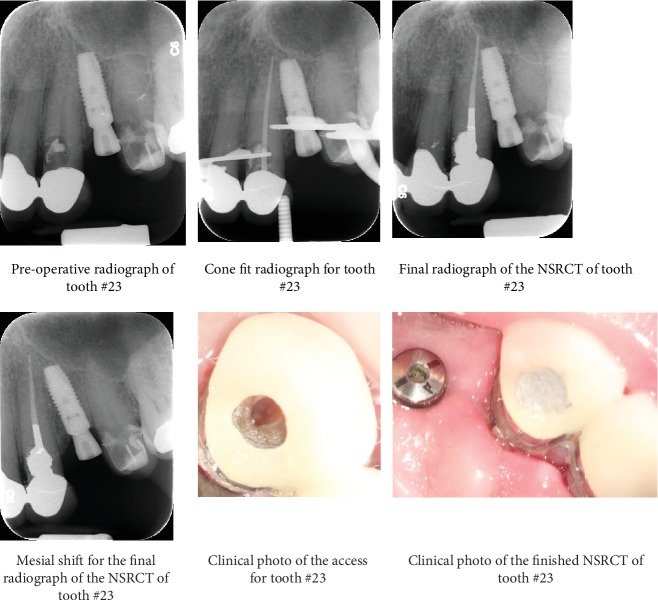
Radiographs and clinical photos showing the steps of nonsurgical root canal treatment of tooth #23 with amalgam post and core buildup.
